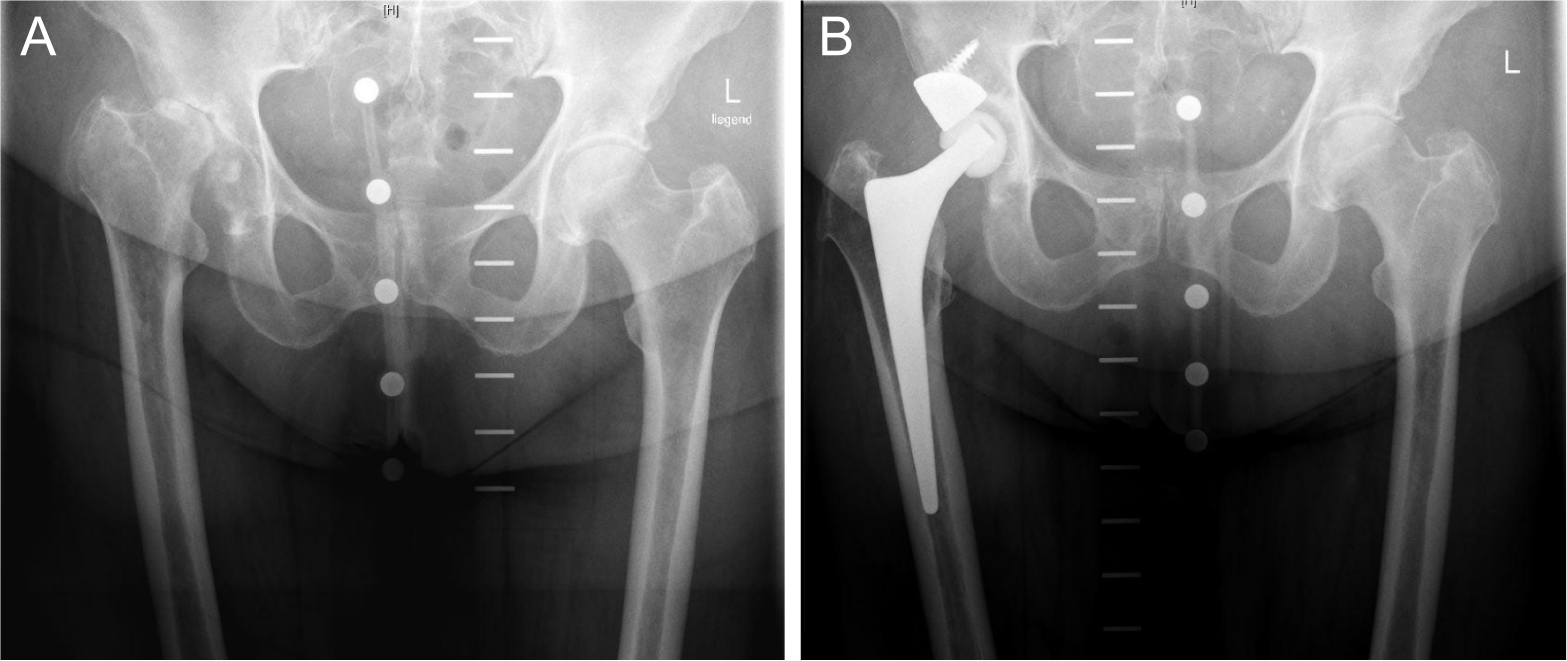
A Preoperative anteroposterior radiograph of the hip of a 68-year-old patient with severe osteoarthritis with advanced acetabular bone loss. B Anteroposterior radiograph of the same patient 6 years after surgery using a modular tantalum TM augment and a cemented PE cup. There are no signs of loosening of one of the components
